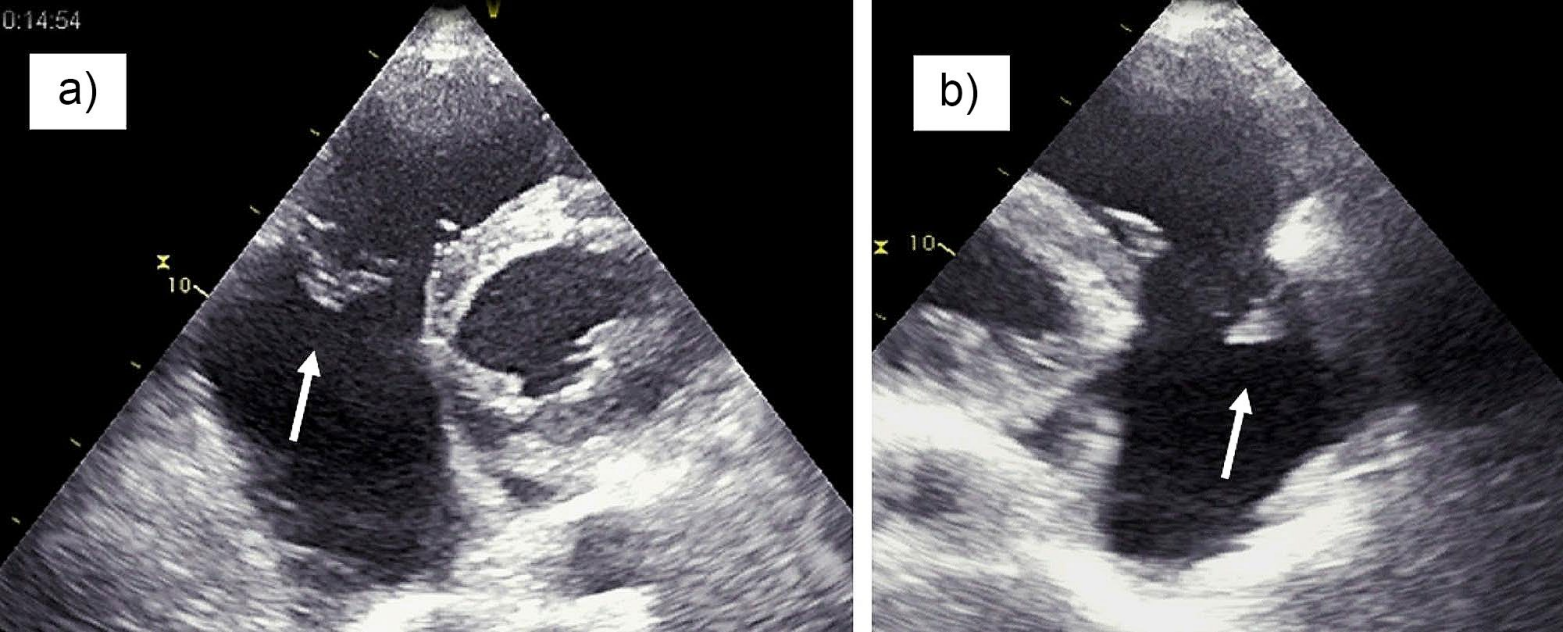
Transthoracic echocardiography showing flail anterior tricuspid leaflet (arrows): (a) parasternal short axis view and (b) modified parasternal long axis view
